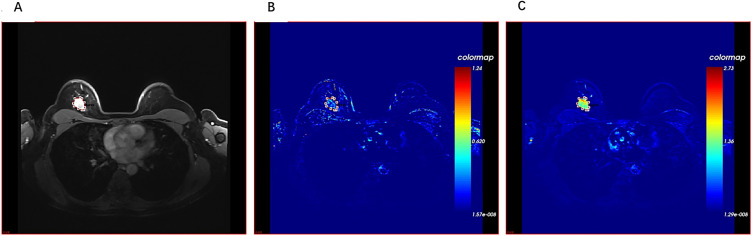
A 51-year-old woman diagnosed with invasive carcinoma of no special type (NST) with negative axillary lymph nodes. (A) Tumor region of interest (ROI) outlined on the early-phase DCE-MRI images. (B) K_ep_ color-coded map. (C) K^trans^ color-coded map. DCE-MRI, dynamic contrast-enhanced magnetic resonance imaging.
